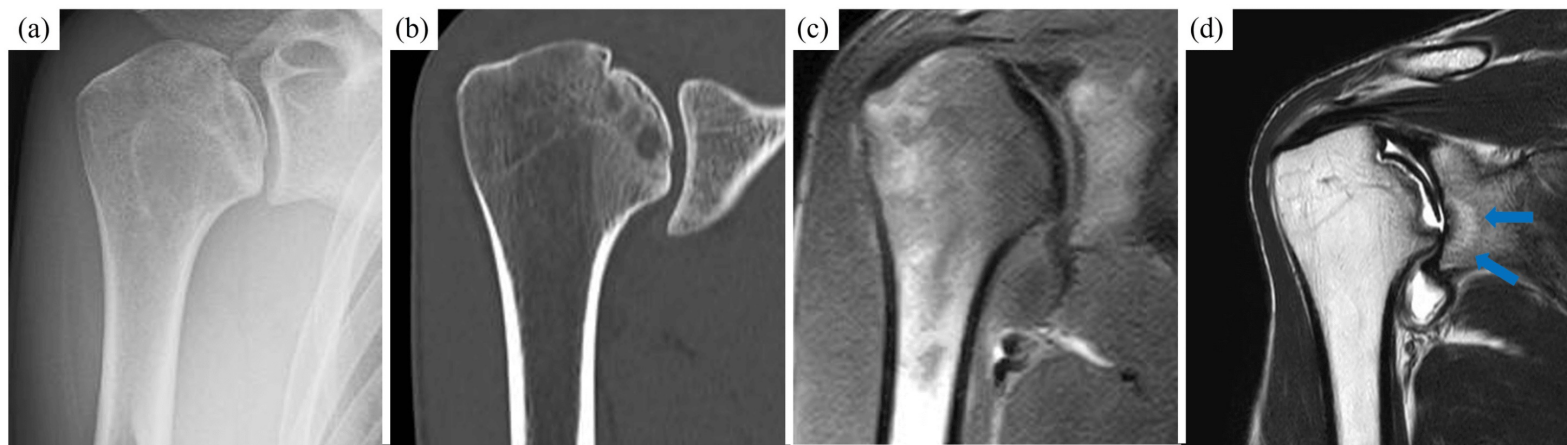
Preoperative images. Preoperative radiograph (a) and CT image (b) revealed collapse and deformation. MRI revealed a depression fracture of the humeral head on the articular surface, bone marrow edema (c), and joint effusion with a high signal area on the side of the glenoid cavity (d, arrows). No obvious low band was observed.
